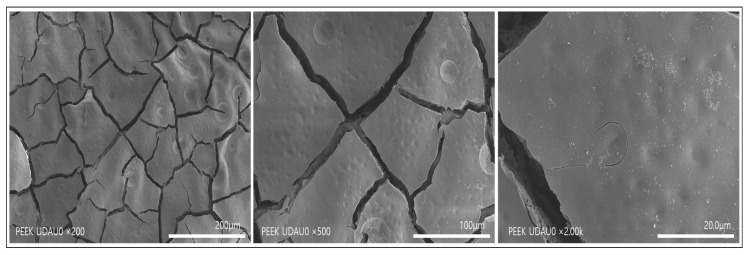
SEM images of PEEK implants treated with a mixed acid of sulfuric and nitric acid for 90 s under ultrasound and then washed with distilled water under mechanical agitation for 6 h.
